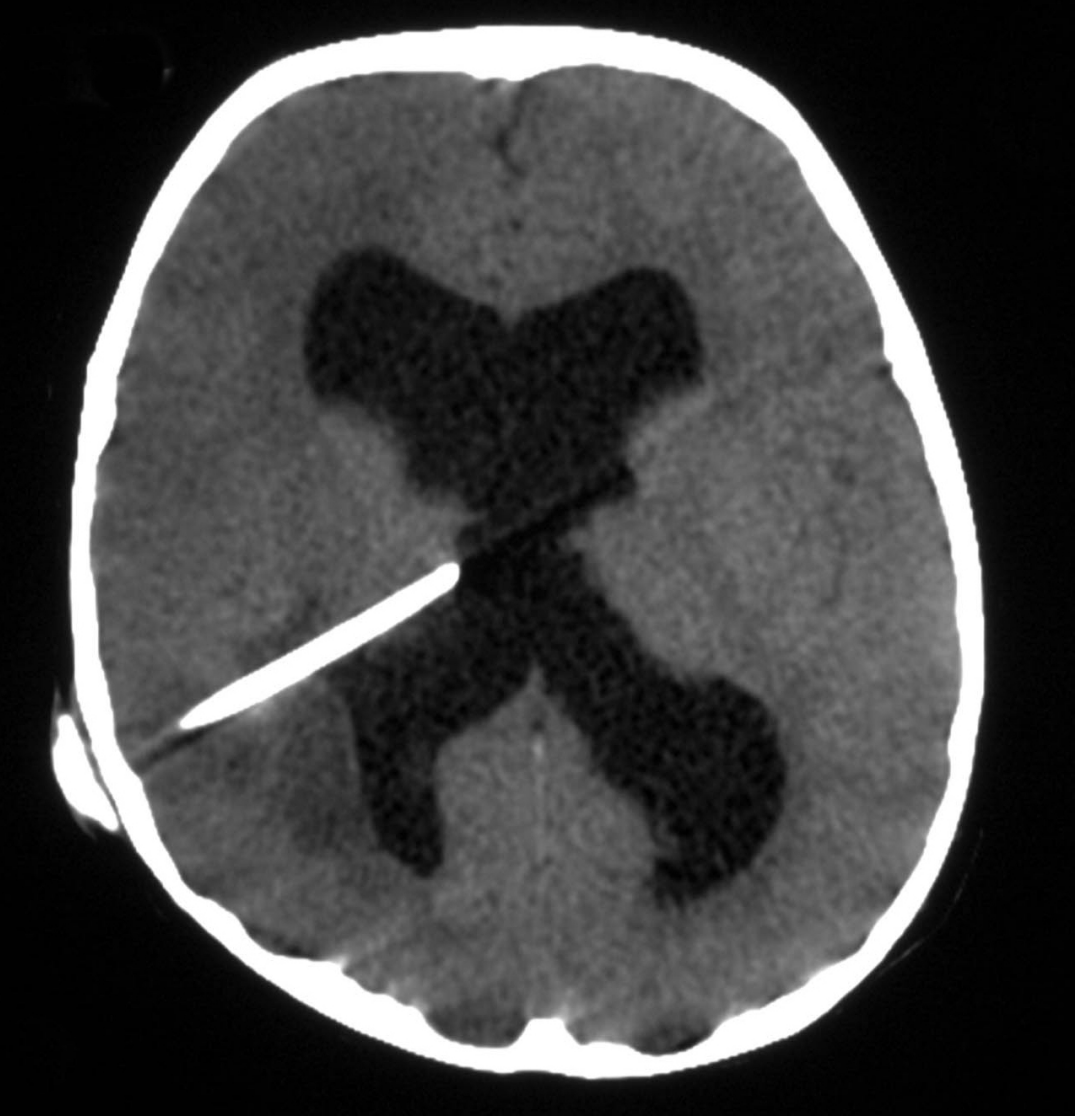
Brain CT (Plain): showing dilated ventricles and right parietal low-density area adjacent to the ventricular catheter and causing slight indentation in the occipital horn.
